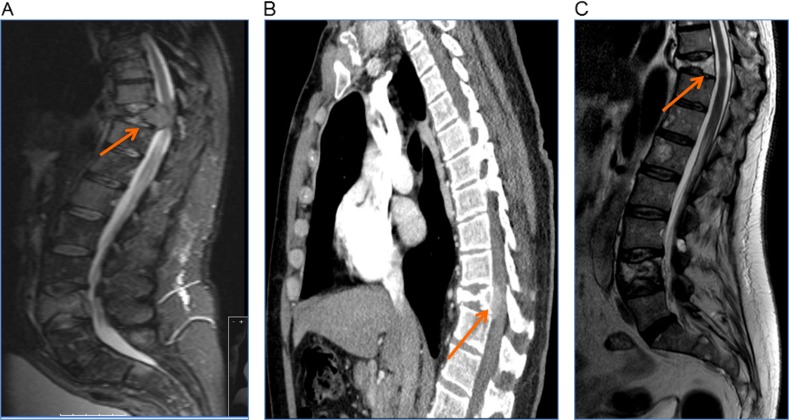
A 57-year-old male presented with bilateral leg weakness (3/5 MRC), sensory disturbances and back pain, catheterised with good anal tone. a Initial MRI revealed T10 collapse with tumour in canal causing spinal cord compression. b Soft tissue CT windowing confirmed that it was soft tissue tumour without bone element in the spinal canal. The patient was treated with dexamethasone and radiotherapy for cord compression, had TLSO brace fitted for relative stability and received 2 cycles of chemotherapy for kappa light chain myeloma. c MRI was repeated for persistent severe back pain (VAS 8/10) and reassessment of cord compression. Clinically power was 5/5 in both legs. The MRI confirmed soft tissue mass response and spinal stability. Patient had cement augmentation with BKP at T10 to relieve the pain and 24 h later VAS was 1/10
